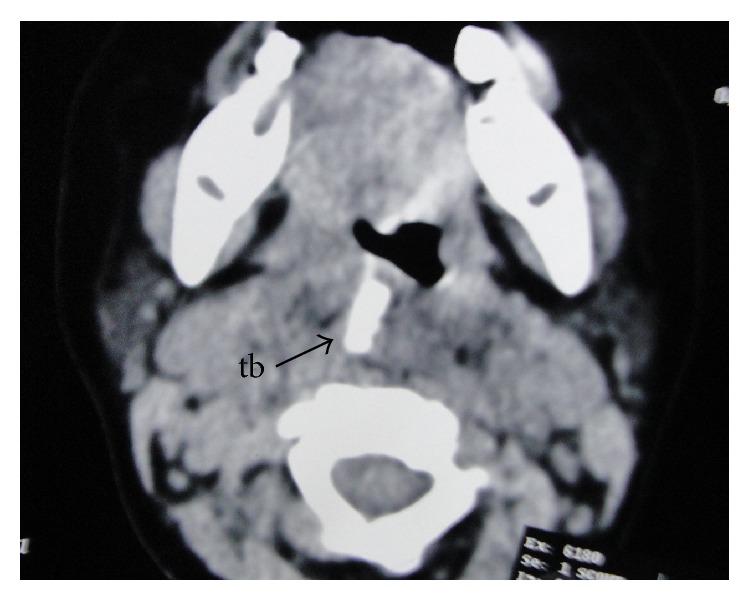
CT scan showing the toothbrush (tb) embedded in the retropharyngeal pace of the oropharynx.
